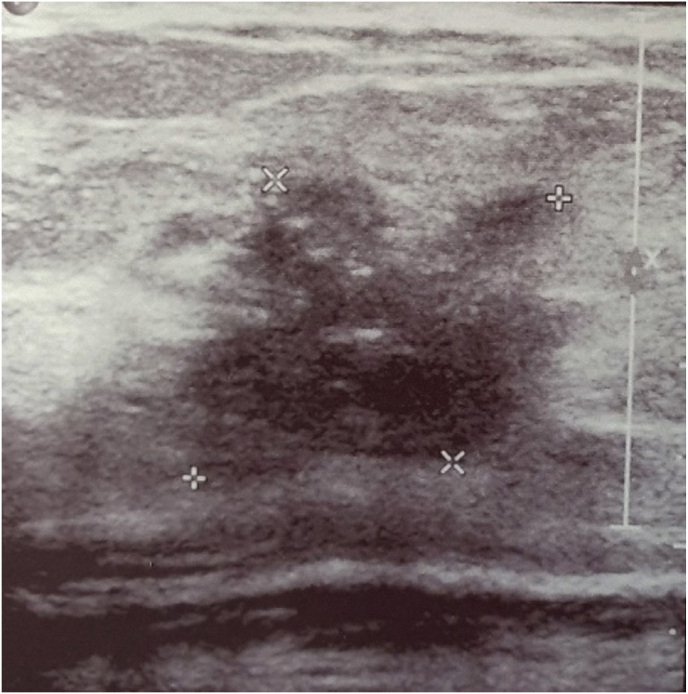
Ultrasound abdomen. Legend: Spiculated, hypoechoic lesion (2.5 × 2.2 × 1.8 cm) with calcification in the right breast.
